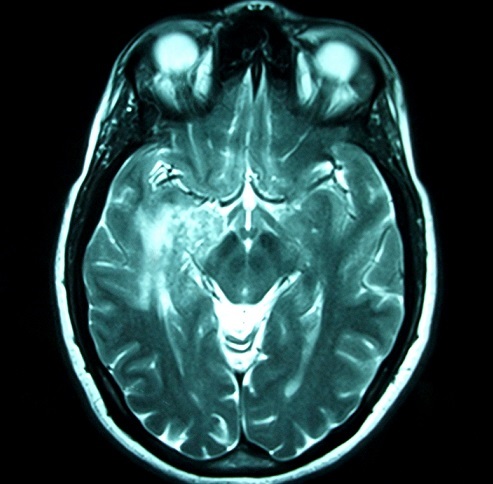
Cranial MRI: T1 post-contrast image in axial view: Enhancing parenchymal nodule involving the left temporal lobe, the lenticular nucleus and the internal capsule
